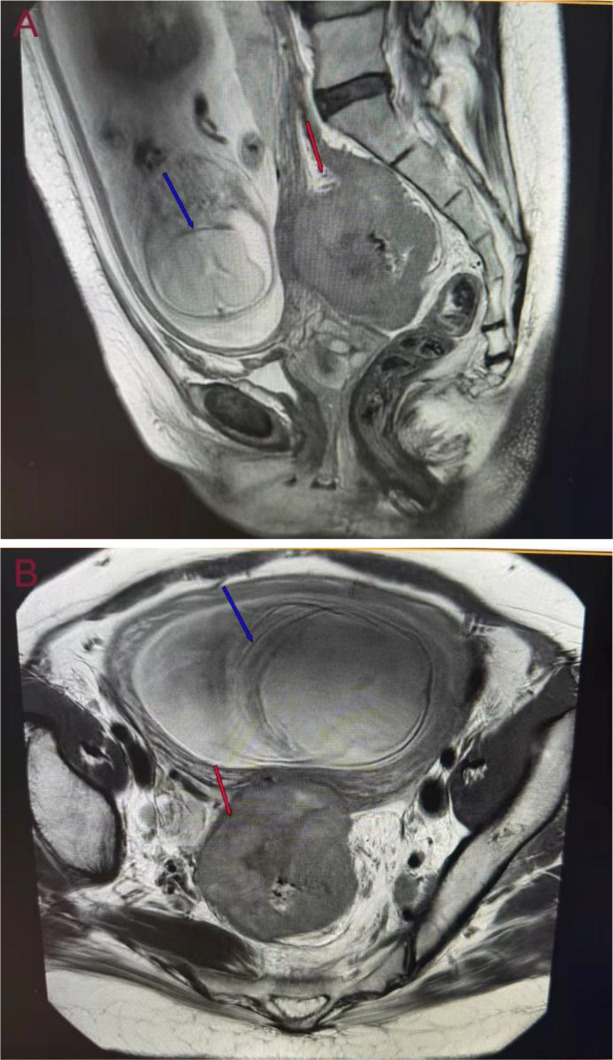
Pelvic MRI findings. (a) sagittal and (b) coronal views showing a rectal mass (red arrow) located posterior to the gravid uterus (blue arrow, fetus). The lesion measured approximately 7.2 × 6.3 × 6.1 cm and was accompanied by multiple enlarged pelvic lymph nodes(cT4N2M0).
